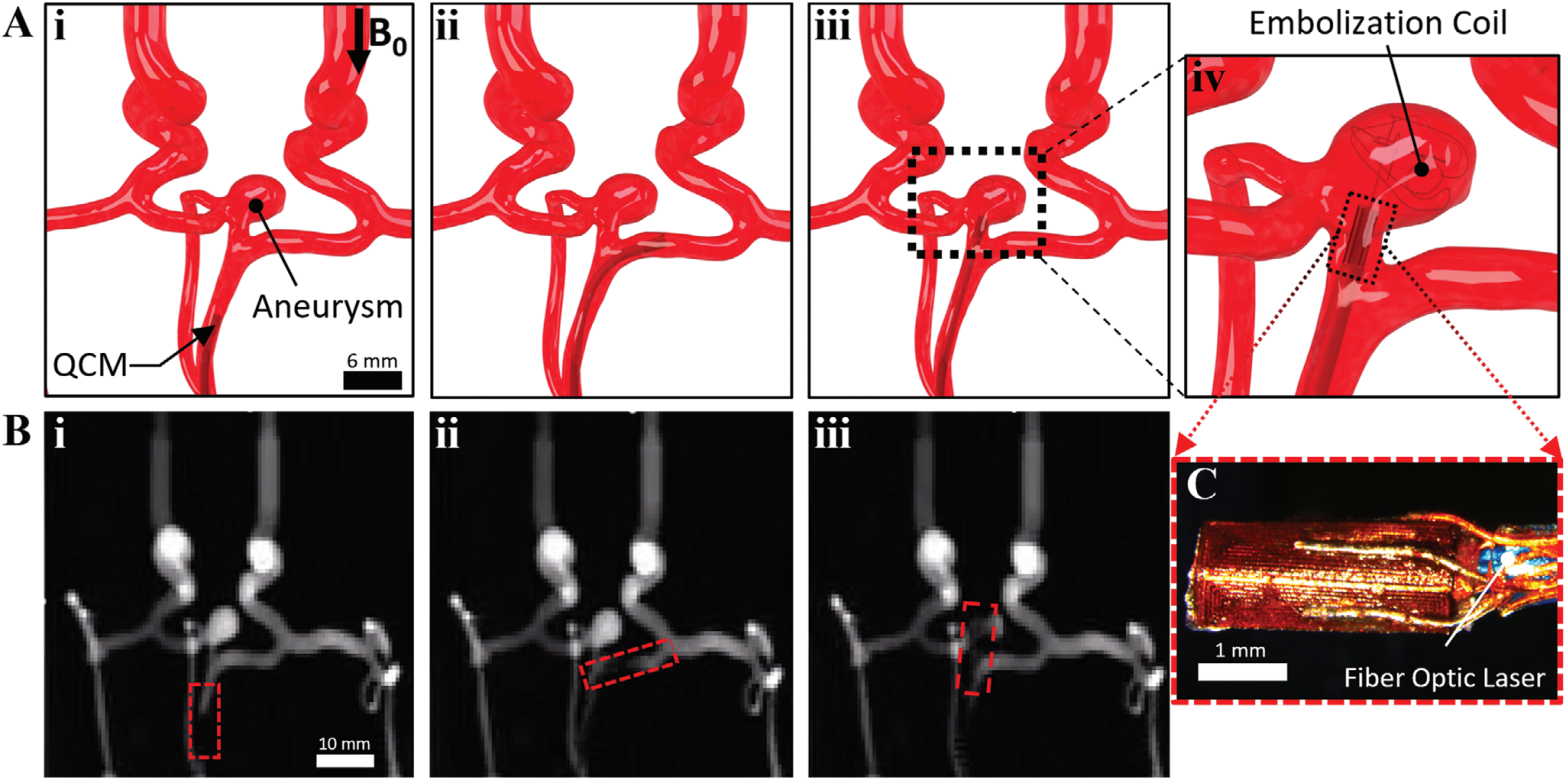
A) The circle of Willis 3D‐computer rendered model and starting position for 3 mm coil length QCM and neuroaneurysm (A.i). A‐ii) QCM taking a right turn at low power. A‐iii) QCM deploying embolization coil to treat aneurysm (magnified depiction in (A‐iv). B) Entire procedure replicated experimentally using MRI‐guidance, where the QCM is tracked by image distortion during Lorentz actuation indicated by the red box. C) Photograph of the QCM with an integrated fiber optic laser.
